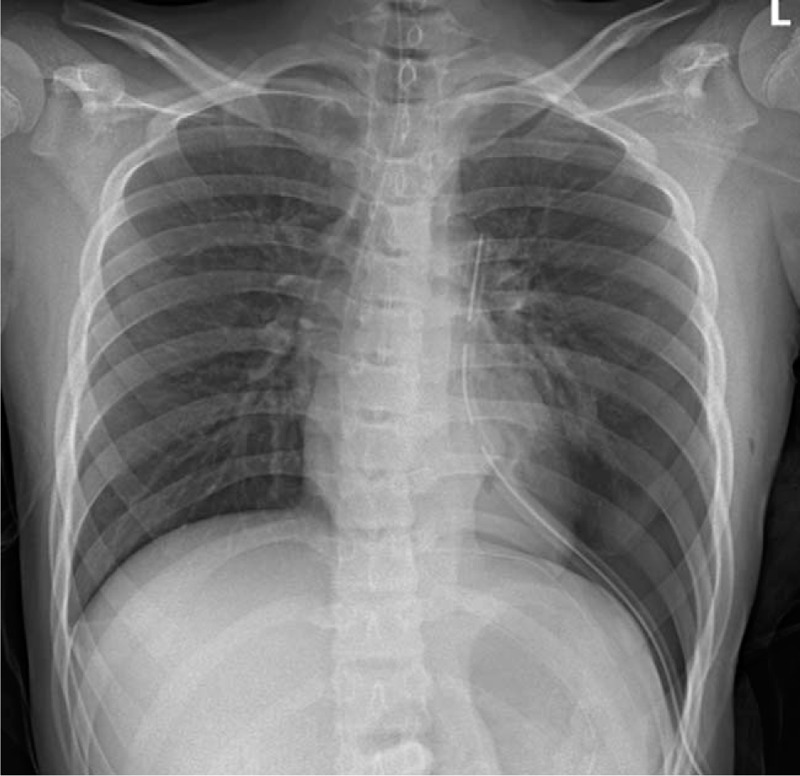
Immediate postoperative chest x-ray after congenital diaphragmatic hernia (CDH) repair shows the clean contour of diaphragm and the normal parenchyma of lung.
